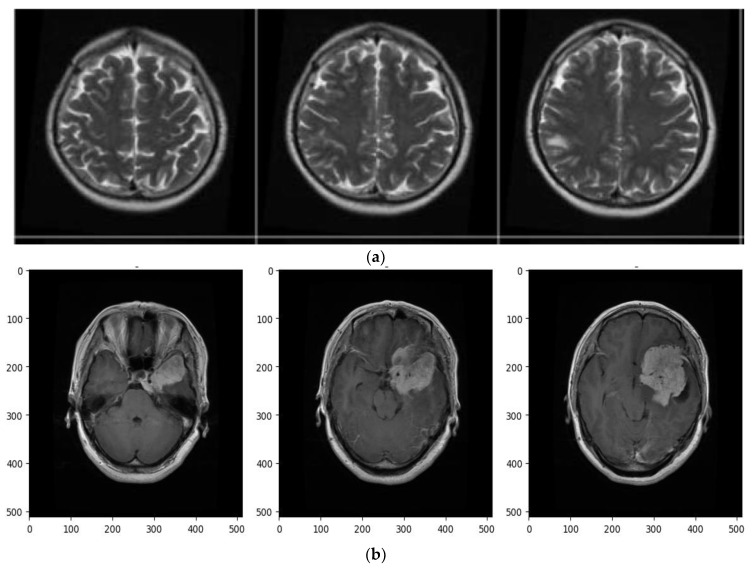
(a) Sample MRI 2D images for normal brain. (b) Sample MRI 2D images for tumor brain.
